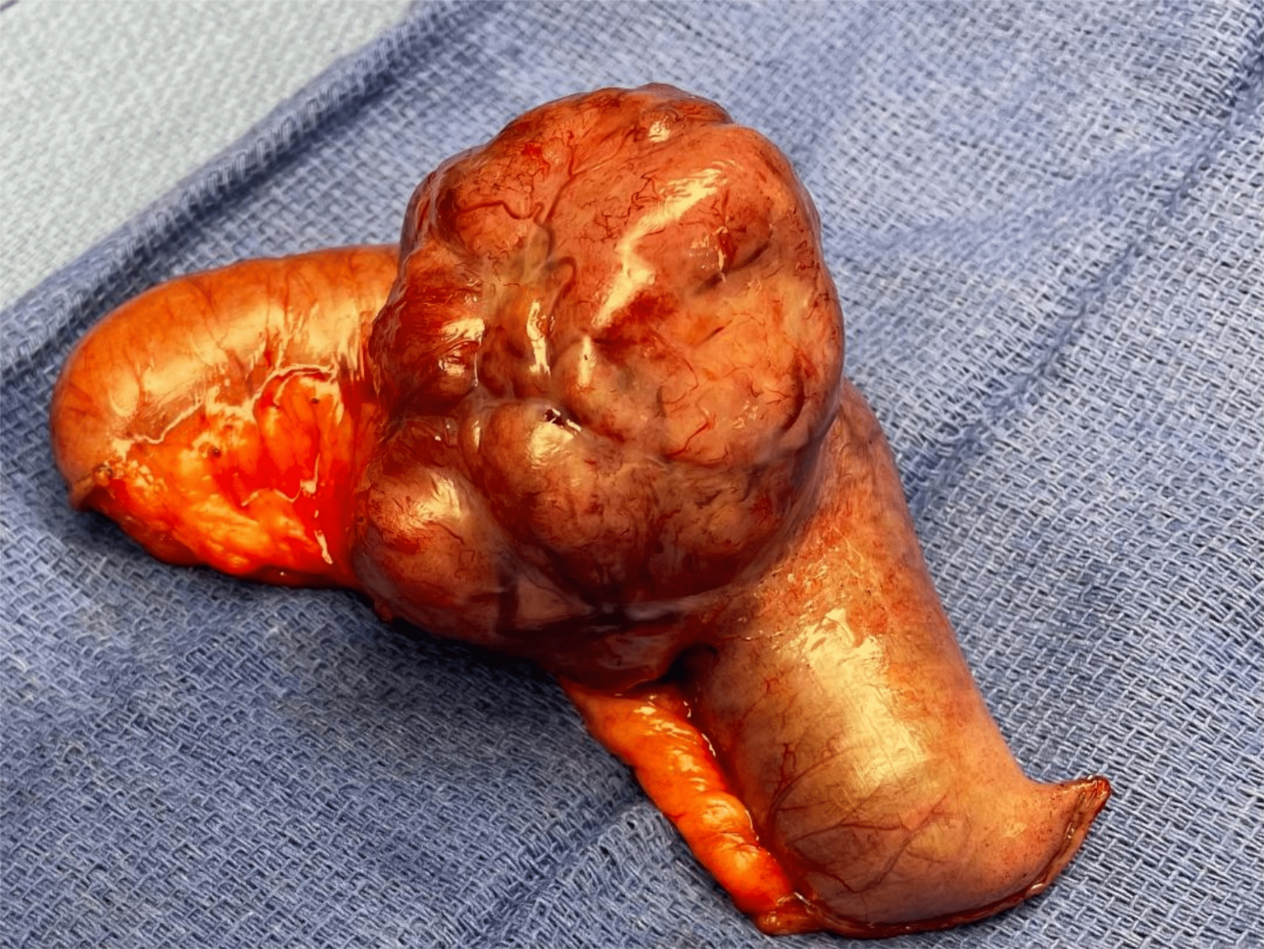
Resected small bowel loop The mass was seen on CT CT: computed tomography
